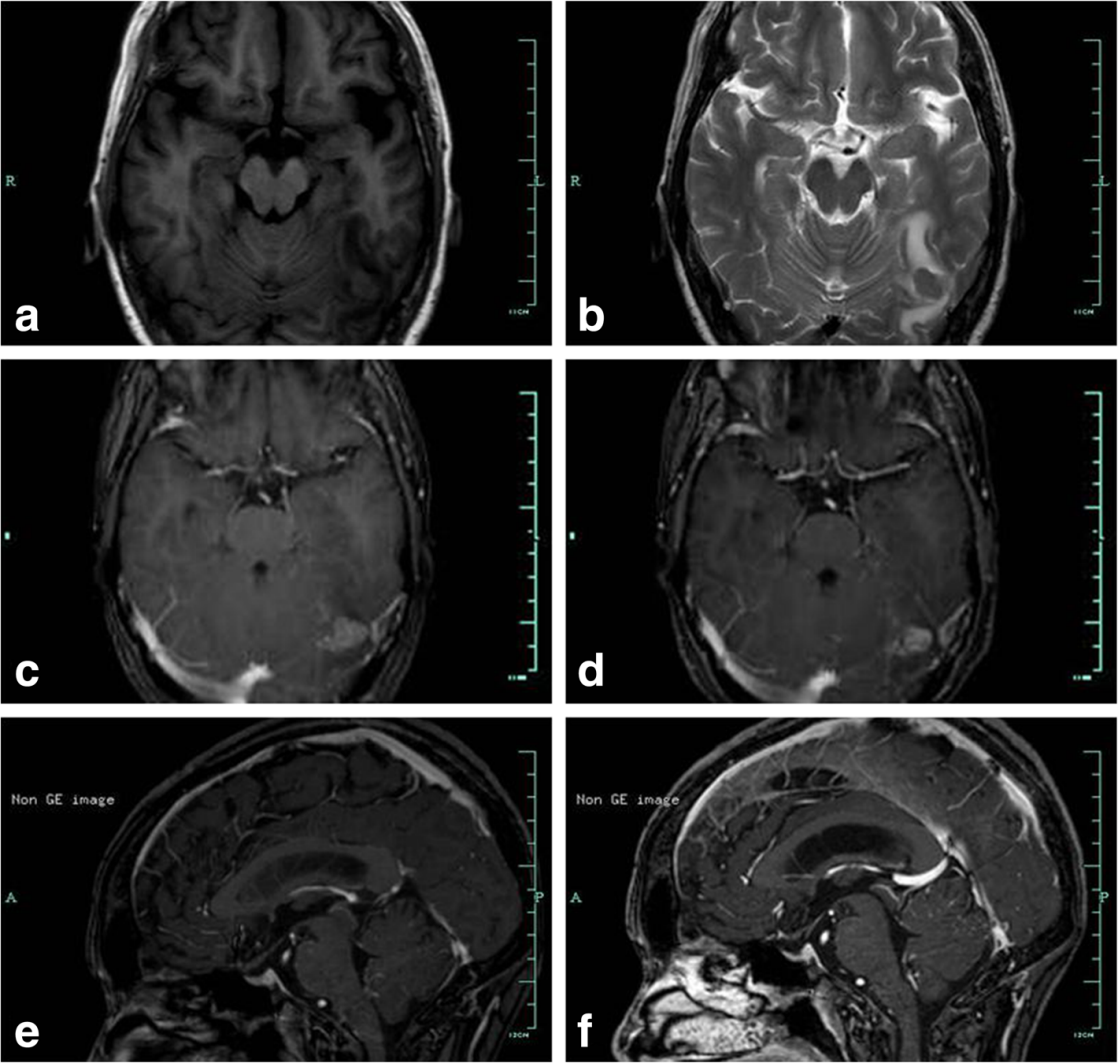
Gadopentetate dimeglumine-enhanced MRI scan shows an ovoid mass with the diameter of 1.6 centimeter in the left occipital lobe. The mass is hyperintense on T1-weighted image (a), iso- to hypointense on T2-weighted image (b), evident homogeneous enhancement on Gadopentetate dimeglumine-enhanced (c) and gadobutrol-enhanced (d) T1-weighted image. Gadobutrol-enhanced T1-weighted image detects nodular enhancement around the cerebellum (f) while gadopentetate dimeglumine-enhanced T1-weighted image shows no abnormality (e)
